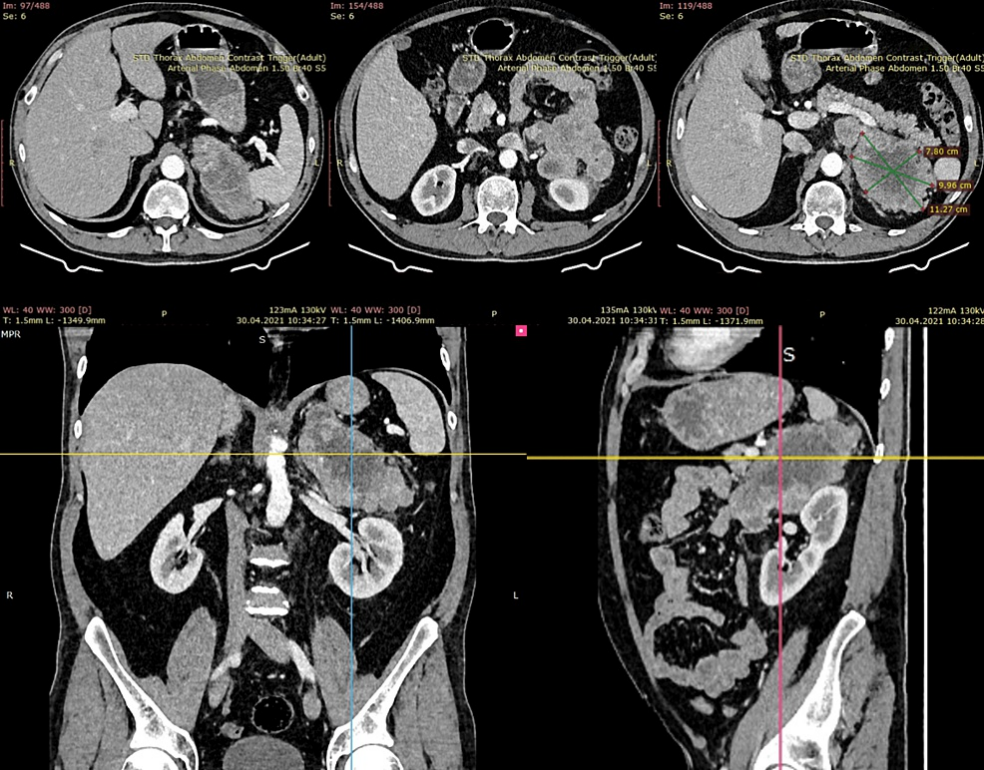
IV contrast-enhanced computed tomography (axial, coronal, and sagittal views) of the retroperitoneal lesion Enlarged formation of 11.6 x 9.0 x 10.3 cm, poorly circumscribed shape, mixed texture (solid-cystic), possibly originating from the small intestine. An expansive growth type, closely adhering to the spleen, upper pole of the left kidney, and left kidney hilum. The tail of the pancreas was not affected by this lesion.
